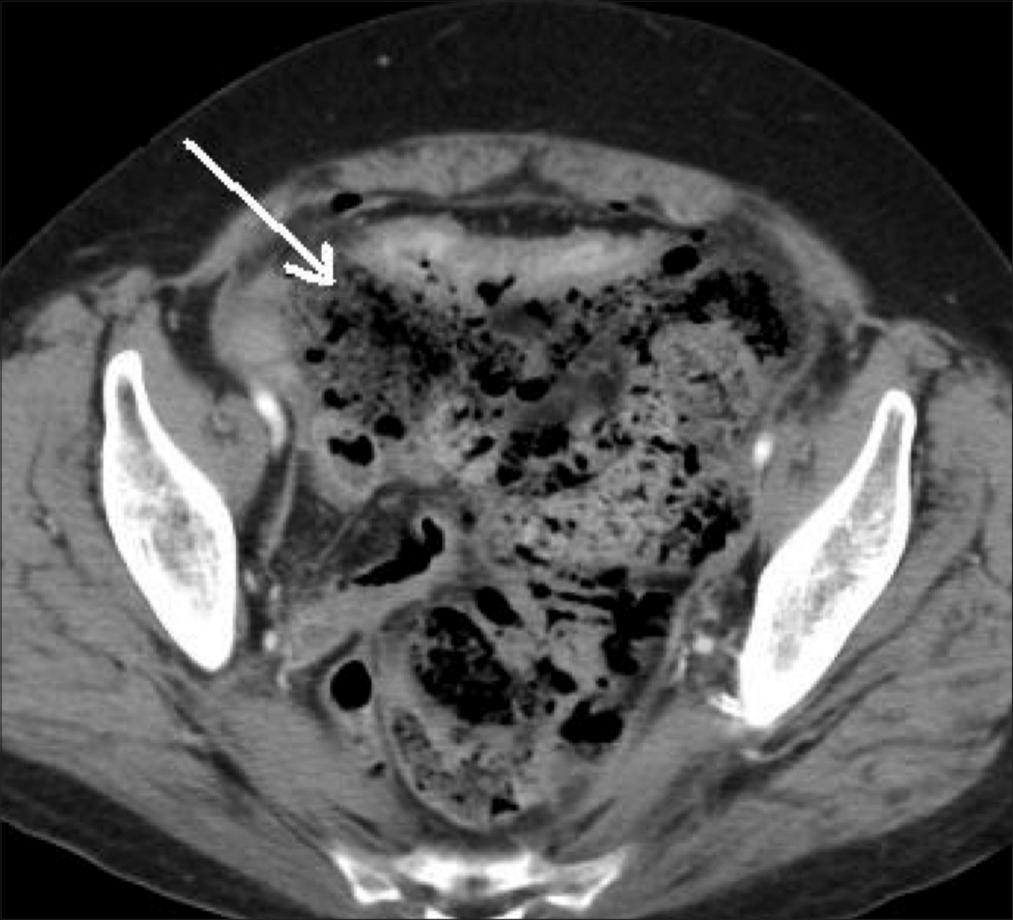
Contrast-enhanced axial CT scan shows extensive extraluminal fecal matter in the peritoneal cavity (arrow) and air loculi, consistent with fecal peritonitis
